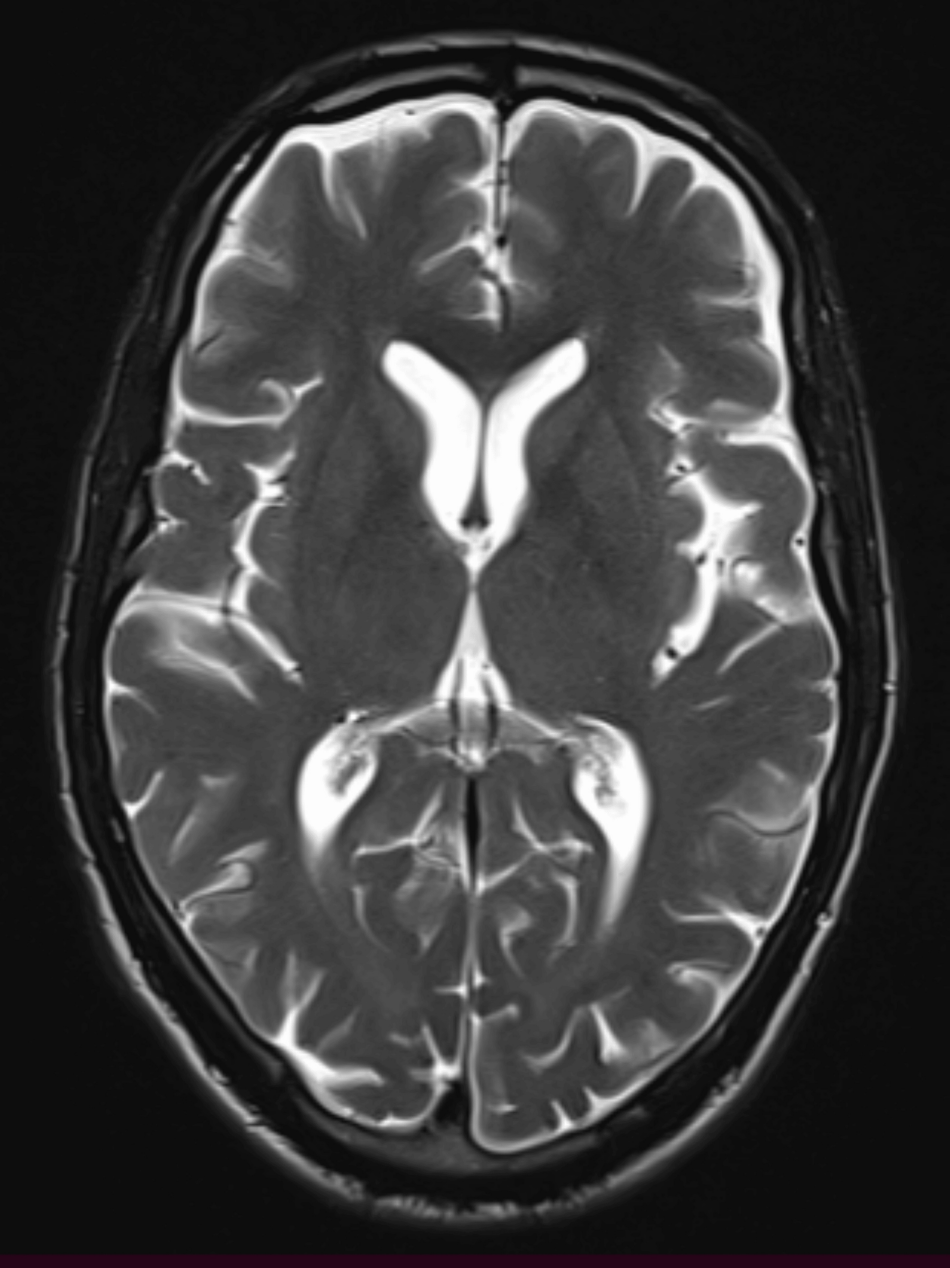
Contrast-enhanced T2-weighted MRI of the brain showing normal findings. MRI: magnetic resonance imaging
